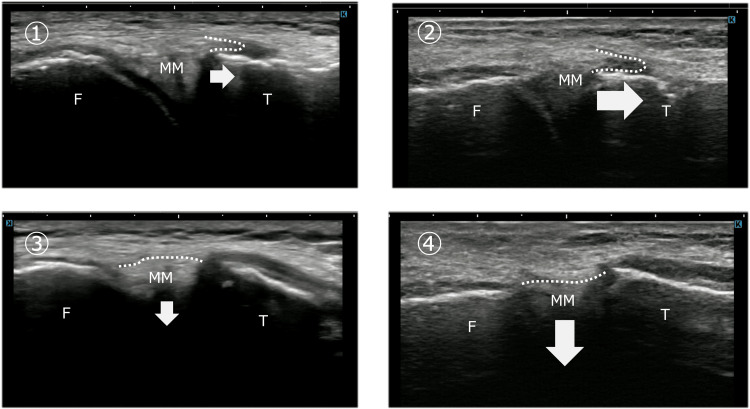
Improvement in medial meniscus mobility after physical therapy in Case 1 Ultrasonographic images demonstrating changes in medial meniscus (MM) dynamics after physical therapy in Case 1. Panels ①→② represent tibial internal rotation, and panels ③→④ represent tibial external rotation. After intervention, the MM demonstrated improved excursion toward both the tibial side and the joint space, indicating restored mobility. These changes were accompanied by resolution of pain during deep knee flexion and cross-legged sitting. F: femur; T: tibia; MM: medial meniscus
